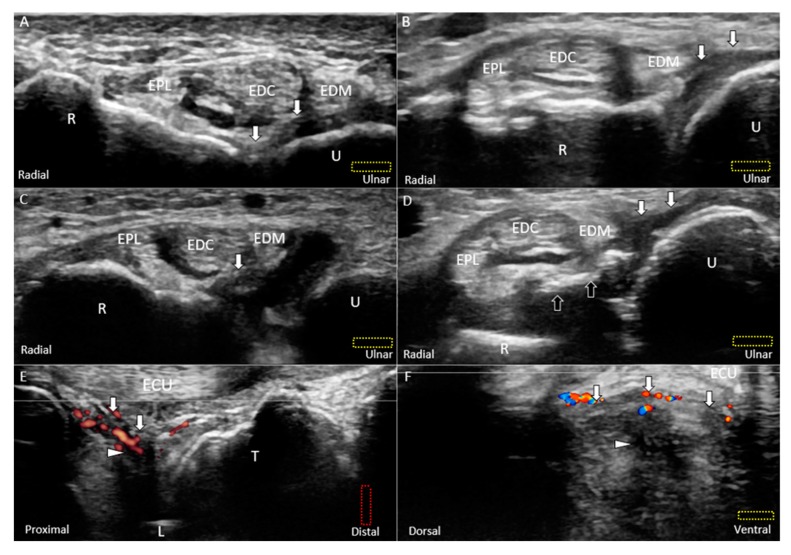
US images (short-axis view) of the (A) normal, (B) partially torn, (C) completely torn, and (D) avulsed dorsal radioulnar ligaments *(white arrow)*. Doppler US imaging of the inflamed dorsal radioulnar ligaments and central tear of the articular disc *(white arrowhead)* using the (E) long-axis and (F) short-axis views. All lesions are categorized under Class 1E injuries. The different color dashed rectangles are in accordance with the transducer positions in Figure 3. US: ultrasound; black arrow: avulsed bony fragment from the radius; U: ulna; R: radius; L: lunate; T: triquetrum; EPL: extensor pollicis longus; EDC: extensor digitorum communis; EDM: extensor digiti minimi; ECU: extensor carpi ulnaris.
